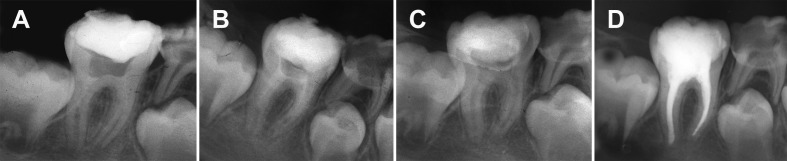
A) Periapical radiograph of first appointment, B) Periapical radiograph 3 months after first appointment, C) Periapical radiograph 6 months after first appointment, and D) Periapical radiograph of final obturation 9 months after first appointment
